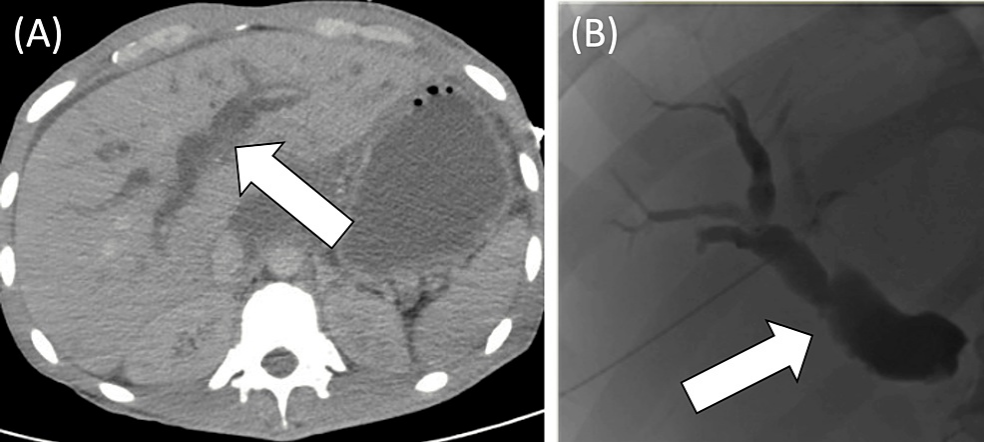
Axial CT scan (A) and cholangiogram (B) demonstrate biliary dilation consistent with cholangitis The white arrows indicate dilated bile ducts. The CT scan (A) was obtained on day 1 when the patient presented to the emergency department, and the cholangiogram (B) was obtained on day 2. Image credits - Dominique Ebedes
